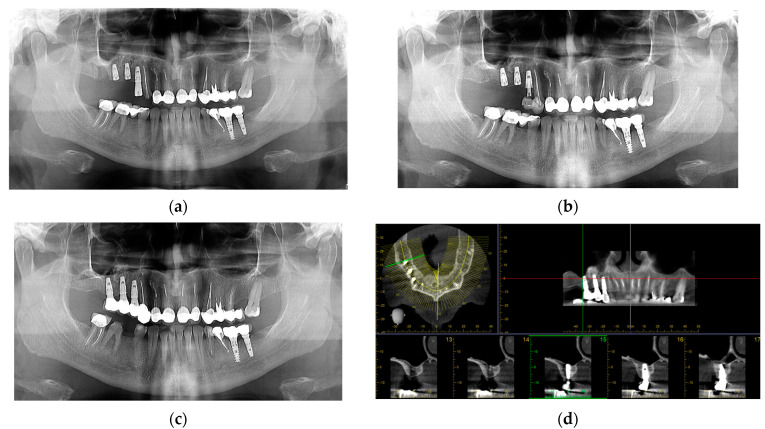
(a) Orthopantomogram immediately postoperative in March 2017; (b) orthopantomogram 6 months later, with temporary crowns; (c) orthopantomogram 62 months later, there were no postoperative complications; (d) CBCT 94 months later without sinus pathology.
